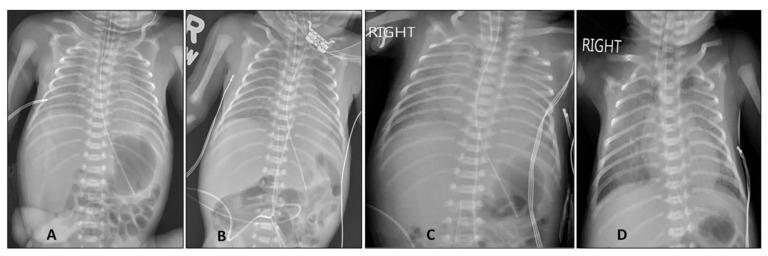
Progression of chest X-ray in the first two weeks after birth. (A). Chest X-ray soon after birth demonstrated low lung volumes and air bronchograms from hyaline membrane disease. (B). Chest X-ray at 48 h of age demonstrated better lung expansion after three doses of intratracheal surfactant administration. (C). The infant was in hypoxic respiratory failure, requiring 100%O_2_ on day 5 after birth. Chest X-ray revealed significant bilateral opacities with low lung volumes. (D). A dexamethasone course significantly improved lung aeration, with decreasing lung opacities on day 12 after birth.
